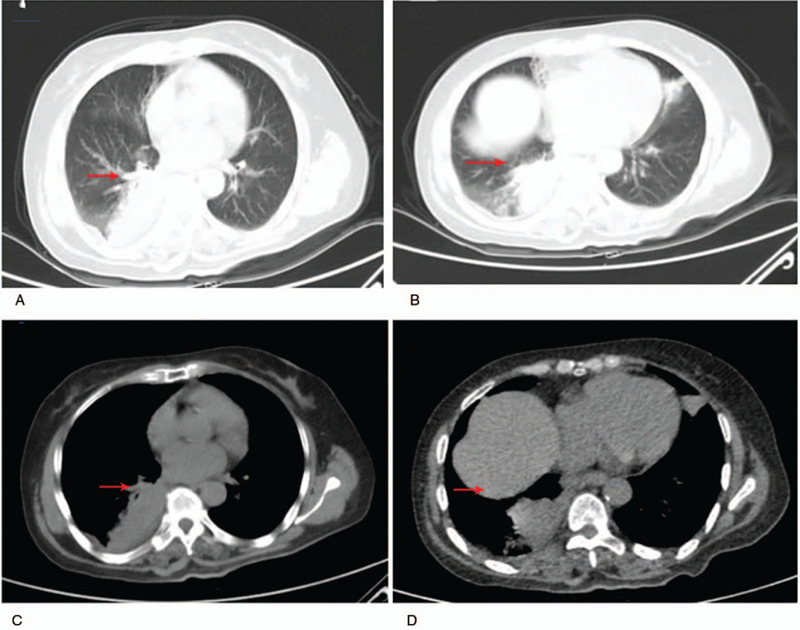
CT (A–D—axial CT images) results showed bilaterally emphysematous lungs with low density para-mediastinal mass in the right upper lobe, obstruction of right upper and middle lobe bronchus with subsequent atelectasis. In addition, vascular invasion was observed in the right upper pulmonary arteries, pulmonary veins, azygous vein and superior vena cava. CT = computed tomography.
